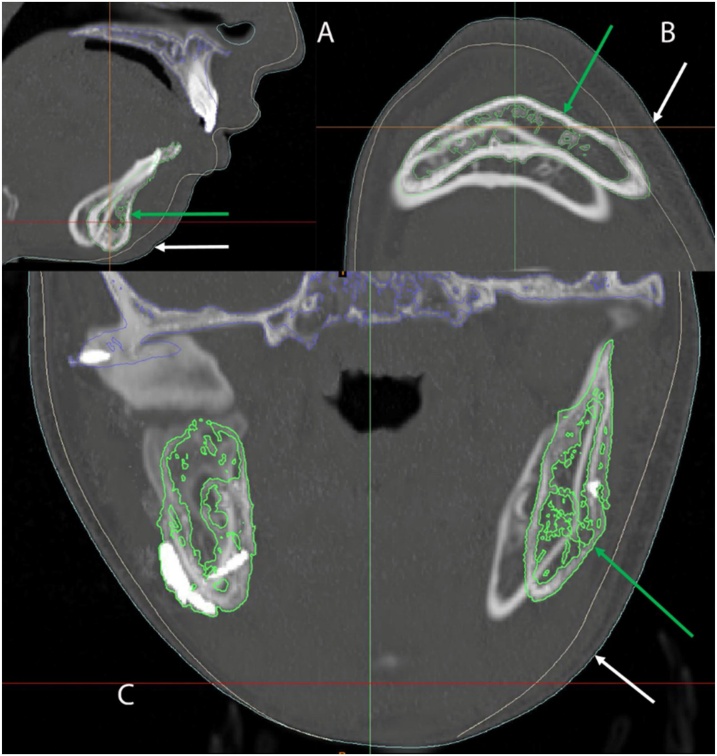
2D CT slices showing the amount of bony and soft tissue correction: A) Sagittal View, B) Axial View, C) Coronal View, *Green arrow representing the postoperative bone, while the white arrow representing the postoperative soft tissue*.
